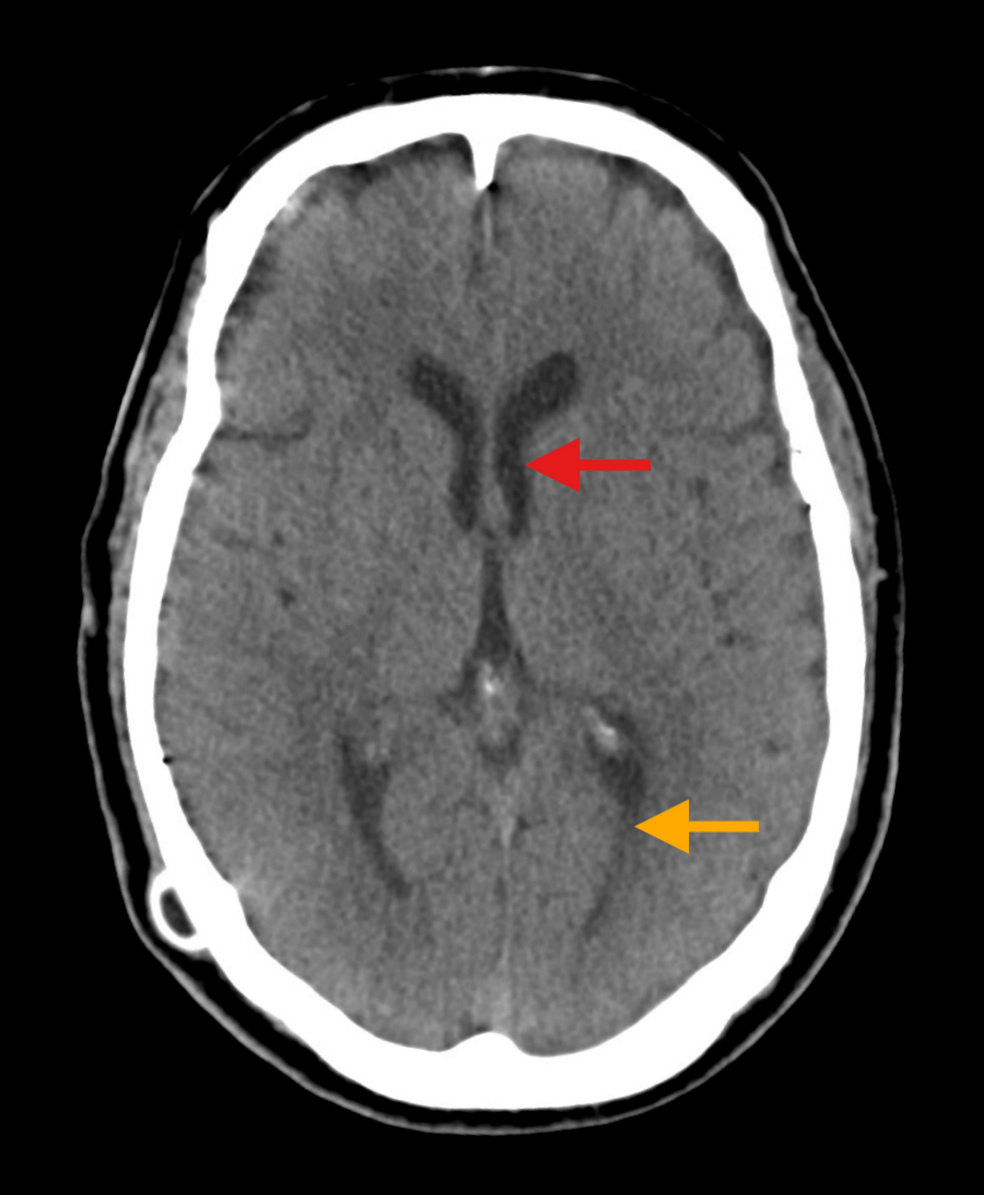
CT brain without contrast Decreased ventricular caliber of the lateral horns: anterior (red arrow) and posterior (orange arrow).
